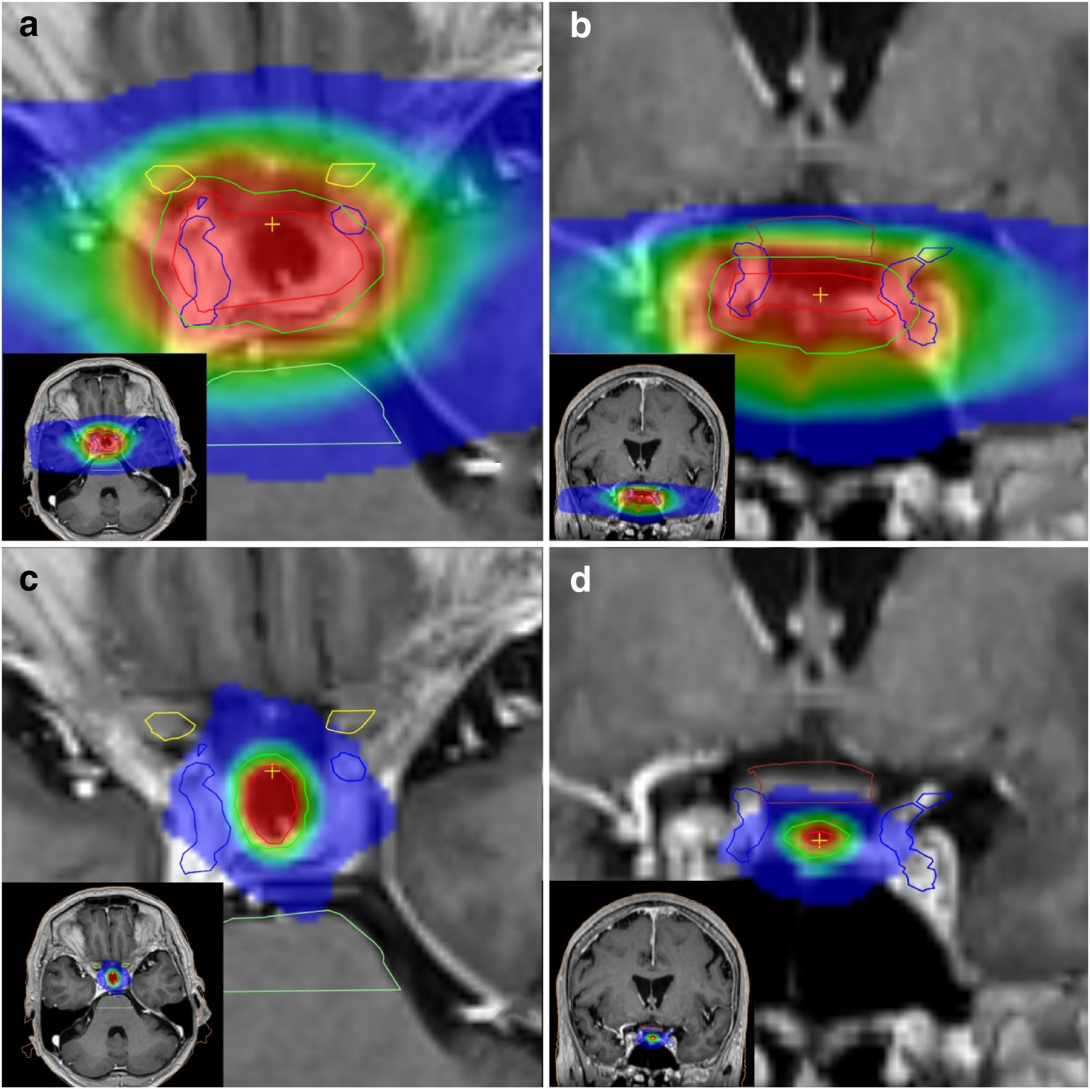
a, b High conformal VMAT planning 28 × 1.8 Gy for a large incomplete resected pituitary adenoma. a Dose projection on transversal contrast enhanced T1 (CE-T1) MR image. b Dose projection on coronal CE-T1 MR image. c, d SRS planning 1 × 20 Gy for a small pituitary lesion. c Dose projection on transversal CE-T1 MR image. d Dose projection on coronal CE-T1 MR image. Contours:* Brown*: chiasm. *Blue* right and left internal carotid artery. *Yellow* optic nerves. *Red* CTV (gross tumor volume). *Green* PTV (planning treatment volume) Dose color wash: *red* >95%, *blue* <10% of prescribed dose. Mean dose right carotid artery for SRS planning: 5.2 Gy (9.0 Gy EQD2). Mean dose right carotid artery for conformal VMAT planning: 49.4 Gy
